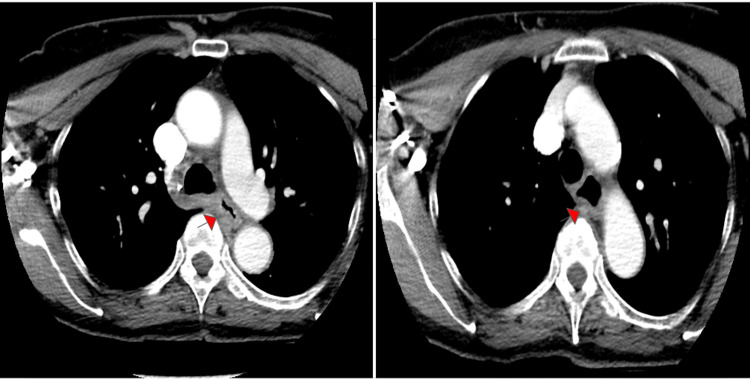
CT neck images Mild mural thickening of the upper thoracic esophagus (red arrows), with compression and collapse of the lumen at the level of the carina by a 3.5 x 2.7 cm mass, which was suspicious for a neoplasm, with differentials including but not limited to esophageal leiomyoma, gastrointestinal stromal tumors, and esophageal carcinoma
